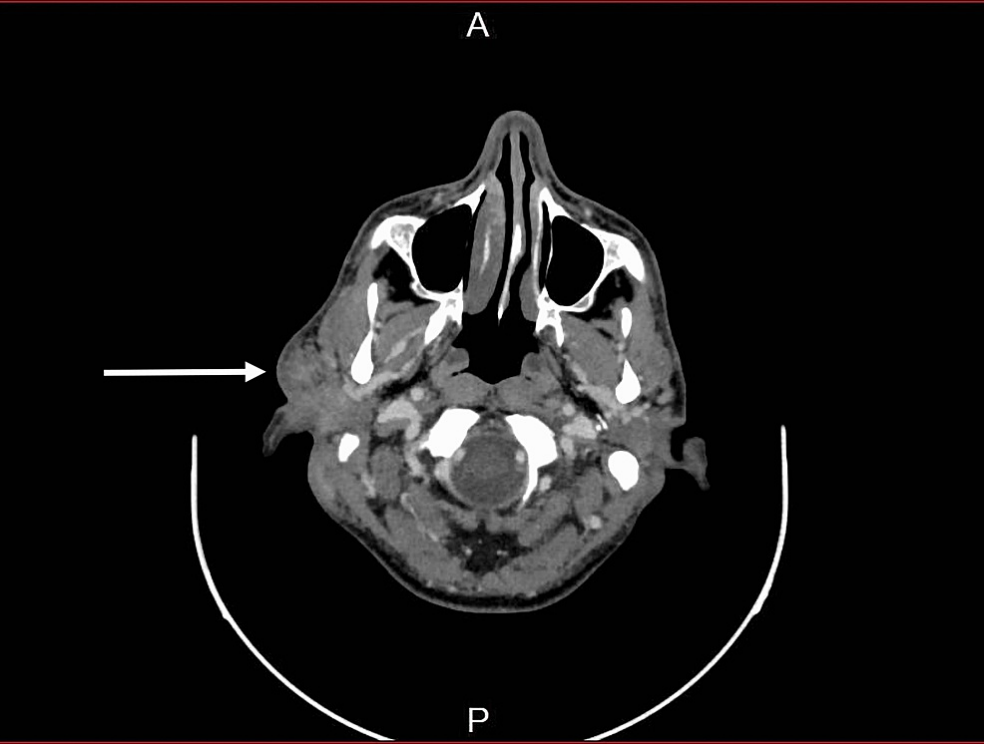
CT showing enlarged heterogeneous right parotid gland CT: computed tomography
